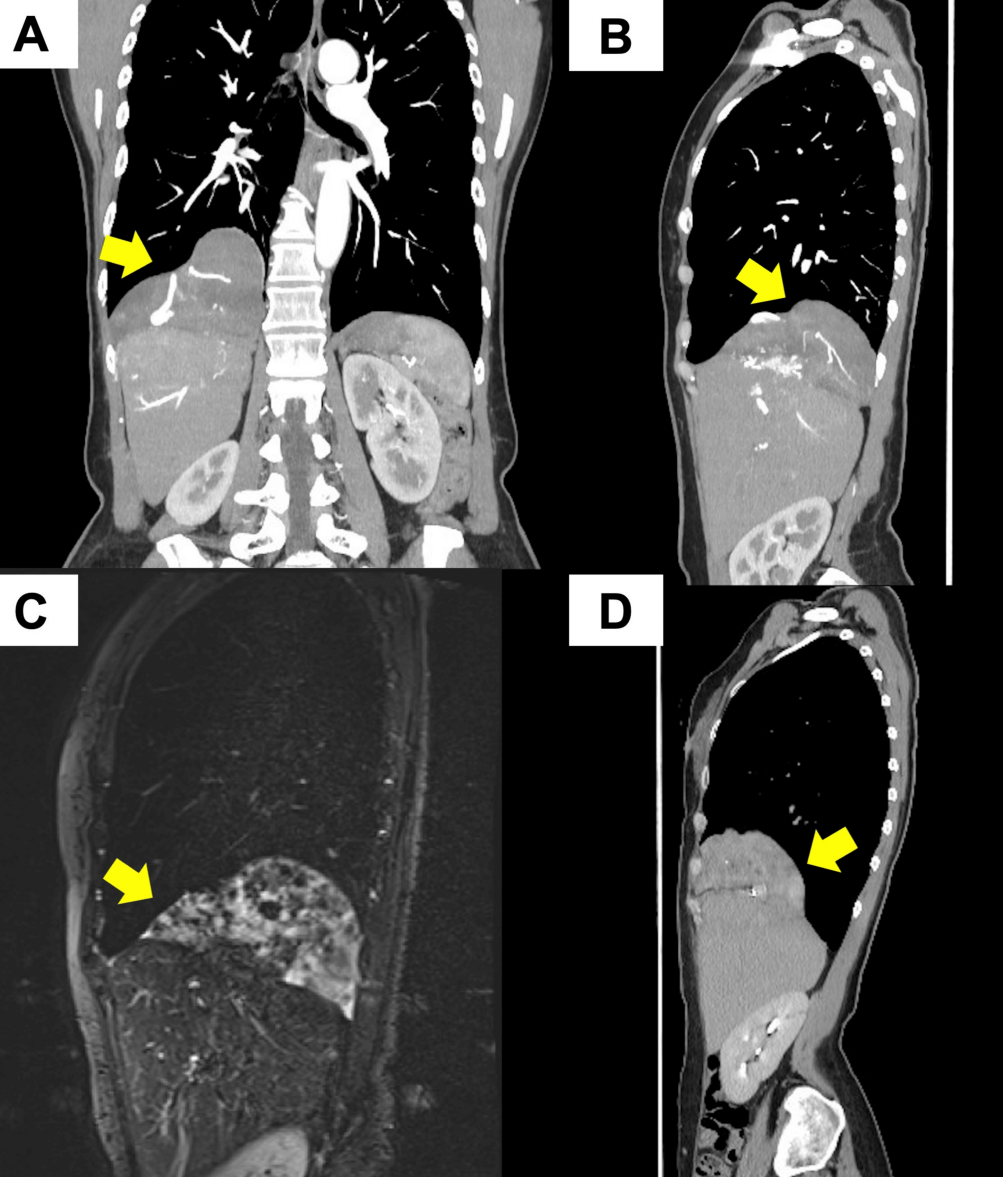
Preoperative imaging findings of the tumor (A, B) Mediastinal coronal and sagittal CT images in the supine position. (C) Mediastinal sagittal short-TI inversion recovery MRI image in the supine position. (D) The tumor is depressed ventrally in the prone position, and there is no invasion of the dorsal diaphragm or chest wall. The yellow arrow indicates the tumor.
